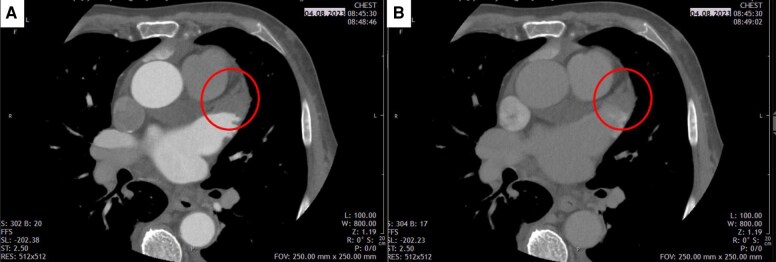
Left atrial appendage thrombus (circle) in early arterial (*A*) and early venous (later) phase (*B*) CCT imaging. CCT, cardiac computed tomography.
